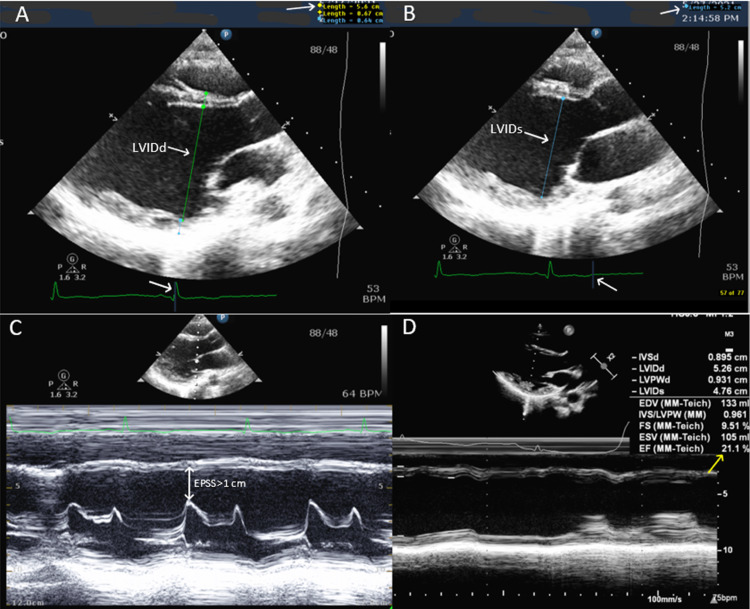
Transthoracic echocardiographic (TTE) images. Image A: shows left ventricular internal diameter end-diastole (LVIDd) 5.6 cm (white arrows) (reference range 3.5-5.6 cm). Image B: shows left ventricular internal diameter end-systole (LVIDs) of 5.2 cm (white arrows) (reference range 2.0-4.0 cm). Image C: shows E-point septal separation (EPSS) >1 cm suggesting a low ejection fraction. Image D: M-mode shows reduced ejection fraction (yellow arrow).
